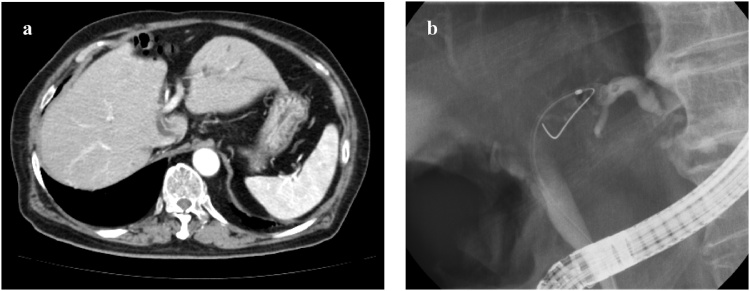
Abbreviations; ERCP: Endoscopic retrograde cholangiopancreatography, CT: computed tomography (a) CT imaging demonstrated dilatation of peripheral side of the left bile ducts. (b) ERCP revealed left-sided bile duct was narrow and the peripheral bile ducts were dilatation. Brushing cytology was performed at the narrow portion, and there was not malignancy in the pathological results.
